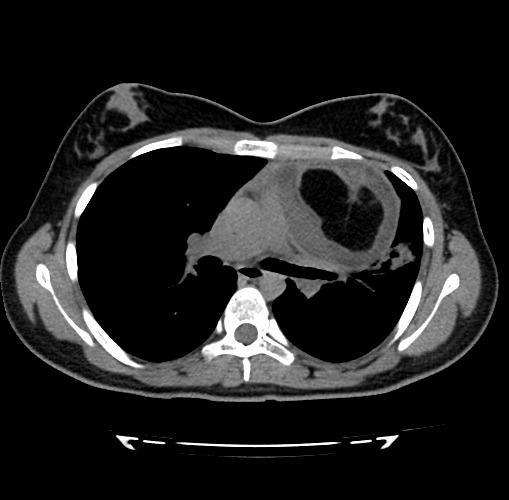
Figure 2: Axial CT scan showing a well-demarcated multi-septated cystic mass compatible with mediastinal teratoma.
